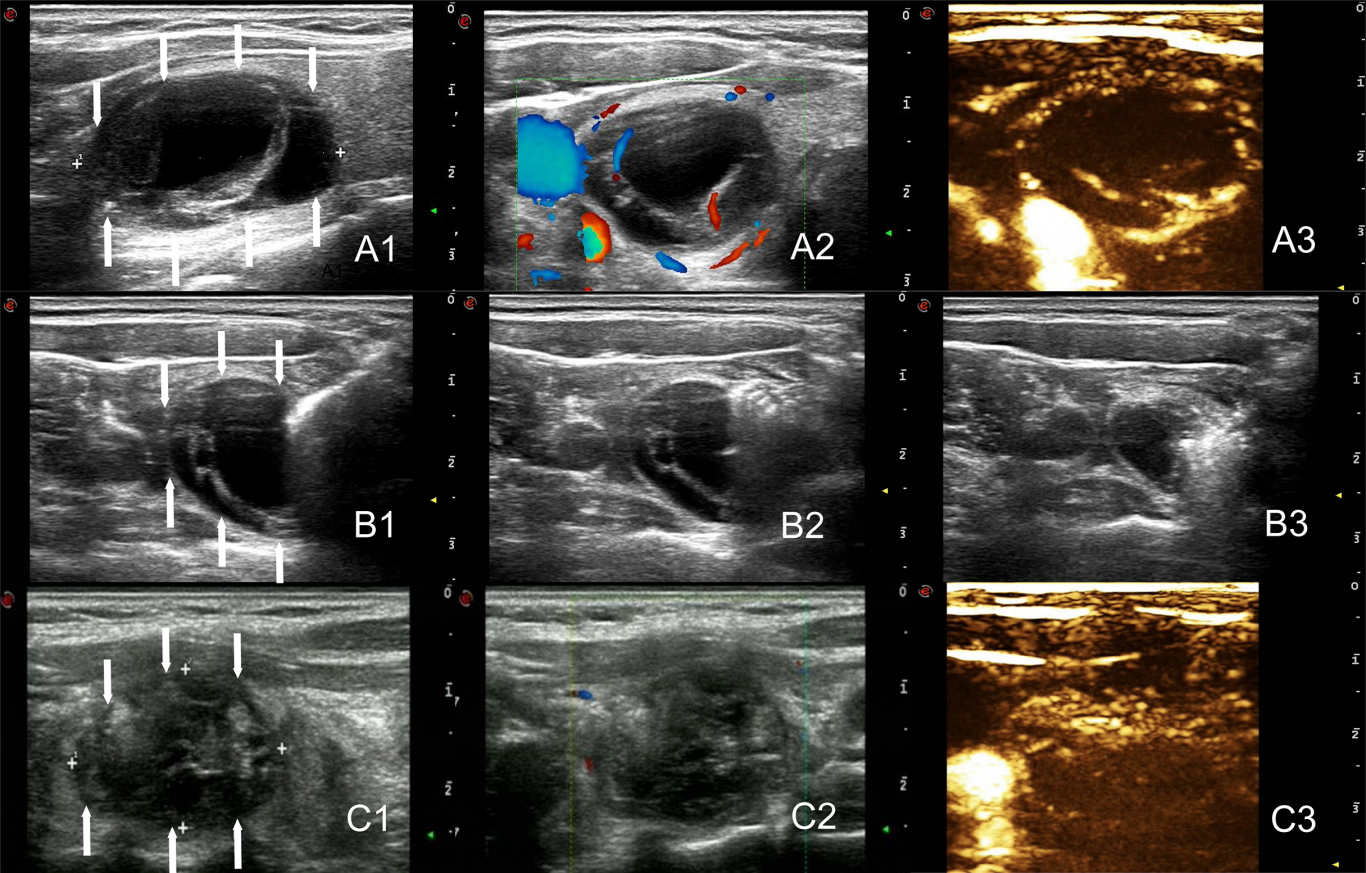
The arrows point to the nodules. Two-dimensional ultrasound, CDFI and CEUS images before treatment of the nodule (Volume = 9.1ml, A1–A3). Images of the tract ablation in group A. One needle tract ablated prior to cyst fluid aspiration, followed by an additional ablation of the entire nodule. (B1–B3). Two-dimensional ultrasound, CDFI and CEUS images immediately after the ablation of the nodule (Volume = 4.4ml, C1–C3).
